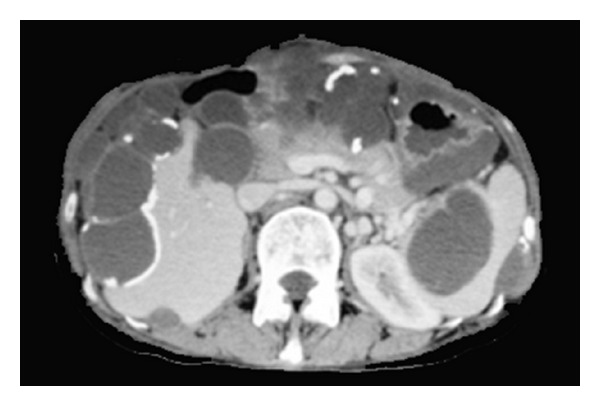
Axial contrast-enhanced CT scan of the upper abdomen demonstrates multiple low attenuated cystic lesions with rim-like calcifications scalloping the liver margin, infiltrating the spleen, and compressing the bowel, pancreas, and left kidney.
